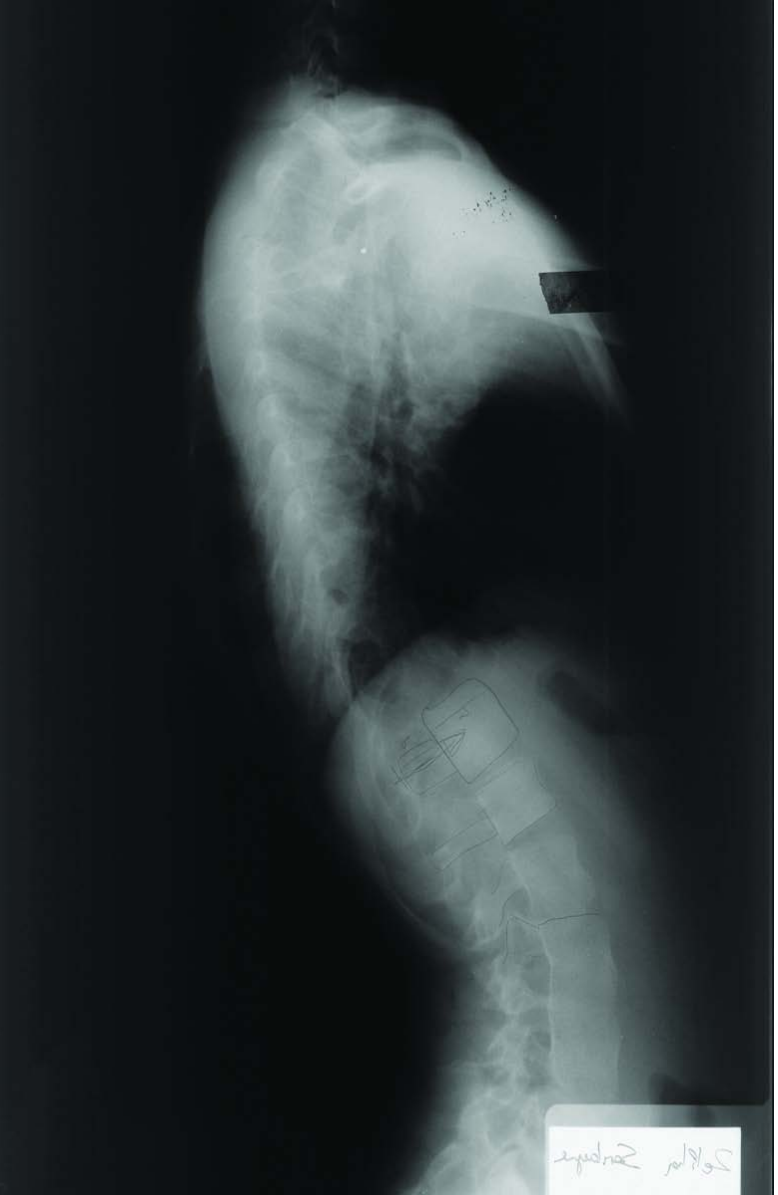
Preoperative lateral radiograph of the patient.
